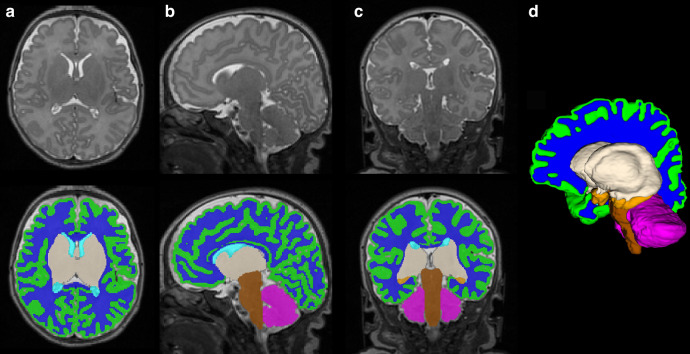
Term equivalent volumetric segmentation images. T2 brain MRI images with corresponding volumetric segmentation in the a axial, b sagittal, and c coronal planes with d 3D volumetric reconstruction. *Green*: cortical gray matter, *blue:* white matter, *gray*: deep gray matter, *tan*: amygdala-hippocampus, *pink*: cerebellum, *brown*: brainstem.
